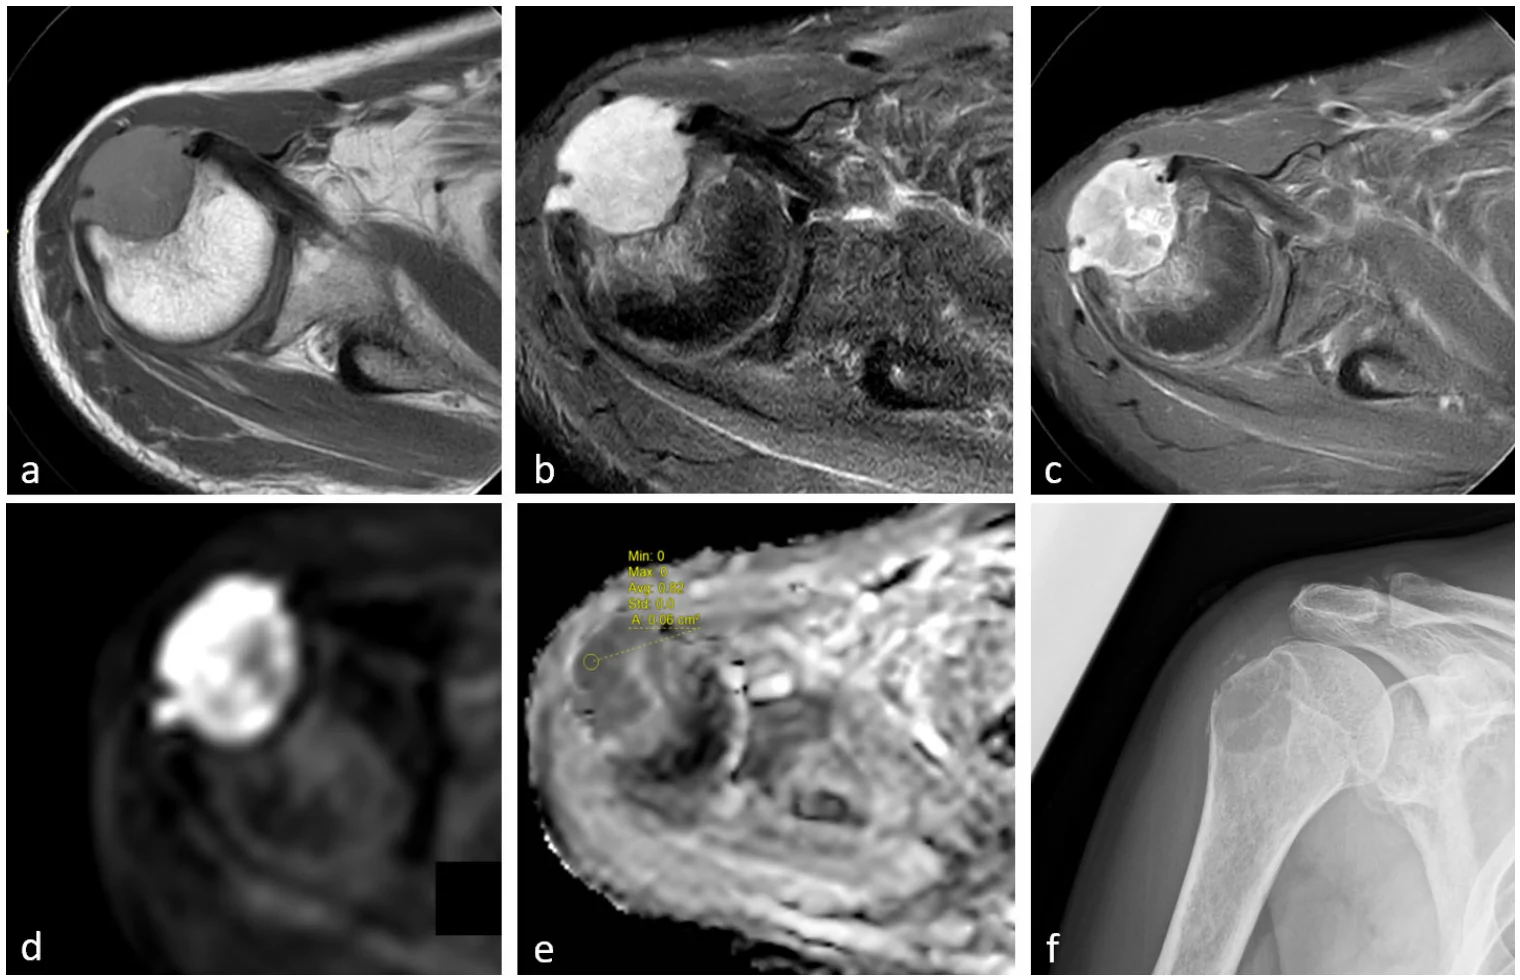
Axial MRI and radiograph demonstrating a metastatic lesion in the humeral head (image-based diagnosis only). The lesion is T1-weighted hypointense (a) and fat-suppressed T2-weighted hyperintense (b) and demonstrates post-contrast enhancement (c), with restricted diffusion on DWI (d) and the ADC map (e). The frontal radiograph (f) confirms a corresponding lytic lesion.
